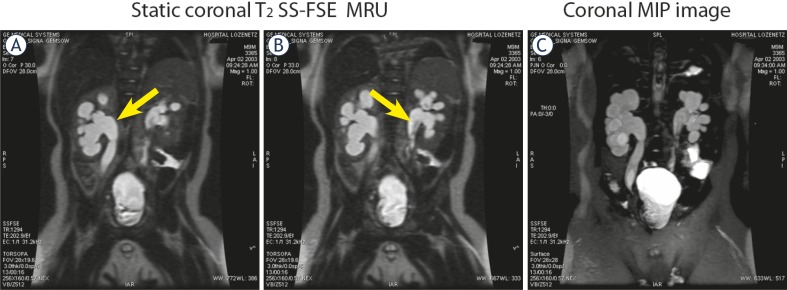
MR imaging of persistent bilateral hydronephrosis and hydroureters in 9-month old boy, following ureterocystoneostomy. A,B. Static coronal T2-weghted MR images using single-shot fast spin echo (SS-FSE). C. Coronal MIP image.
